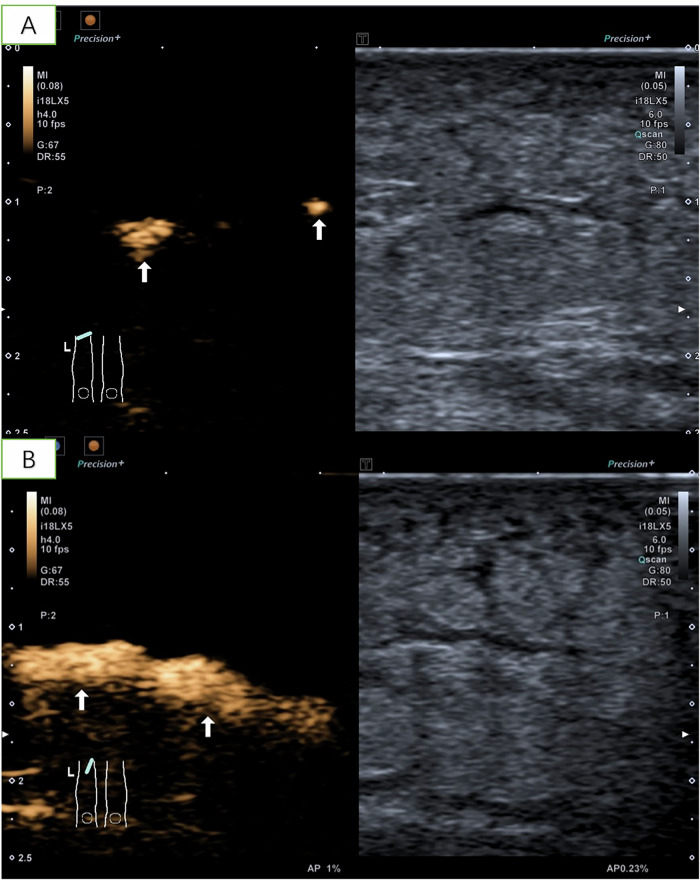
A CEUS shows that lymphatic vessels are round-like structures that appear in the superficial fascia layer in the transverse section. B CEUS revealed linear hyperenhancement with contrast agent bubbles accumulating inside the lumen of the lymphatic vessel along the axis. (white arrow). CEUS, contrast-enhanced ultrasound
